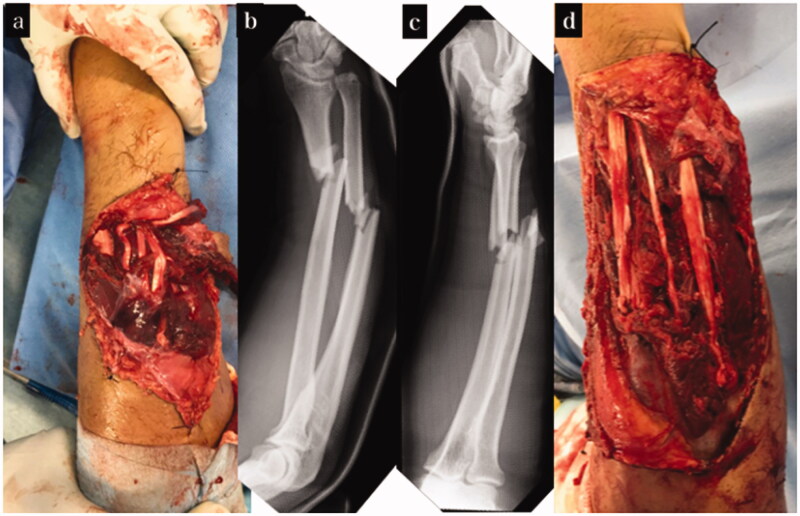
Treatment course of case 1. (a) Appearance on arrival at our hospital. (b,c) X-ray on arrival at our hospital. (d) Appearance after tendon reconstruction using pull-in sutures.
